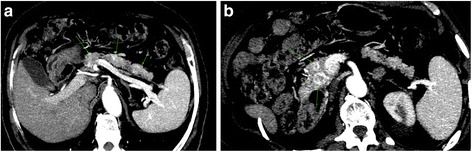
CT findings of the pancreatic metastases. a Body lesion with central necrosis and enhanced rim. b Homogenous hyper vascular head lesion
